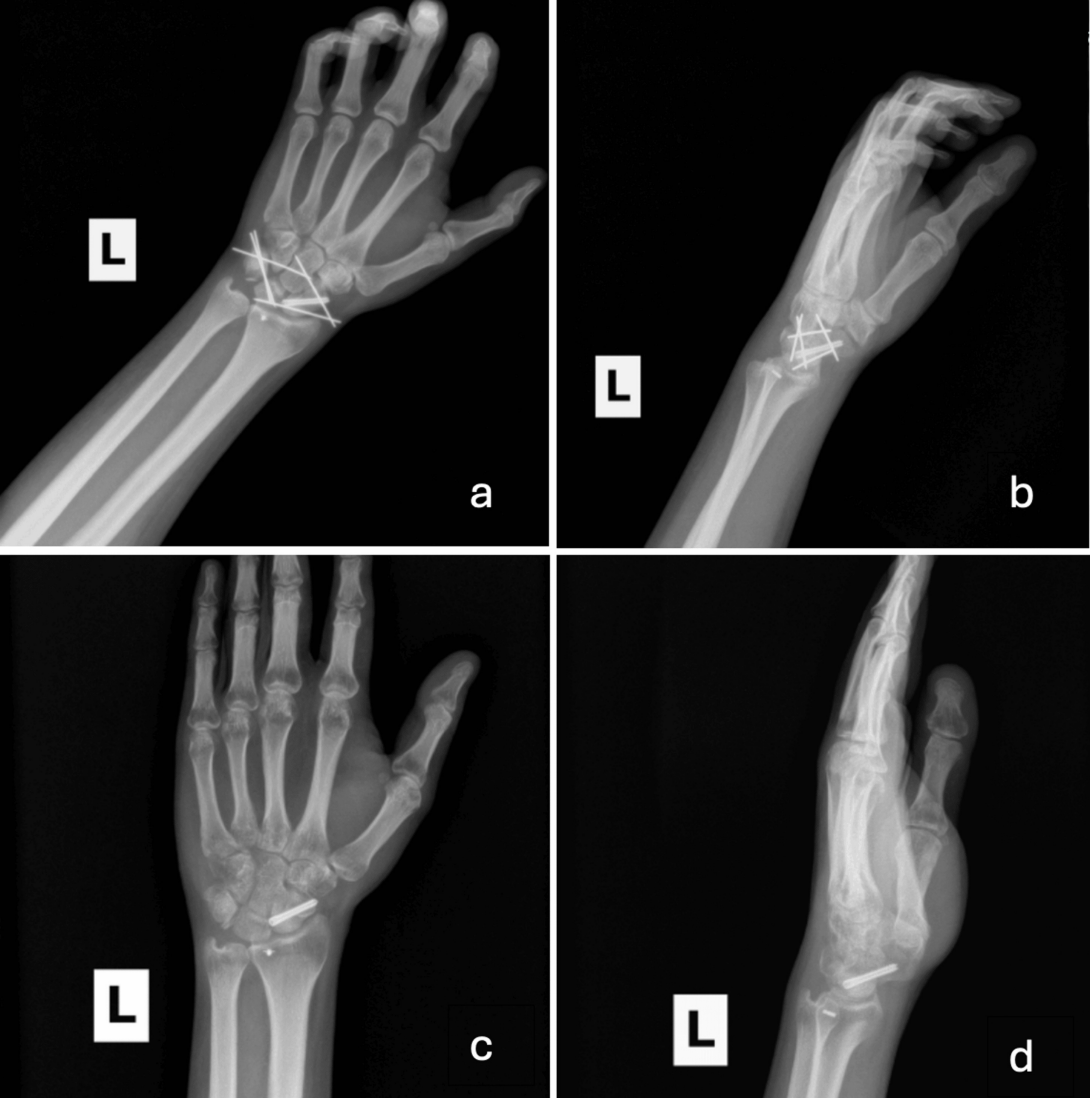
Posteroanterior (a, c) and lateral (b, d) view of pre- and post-hardware removal radiographs.
